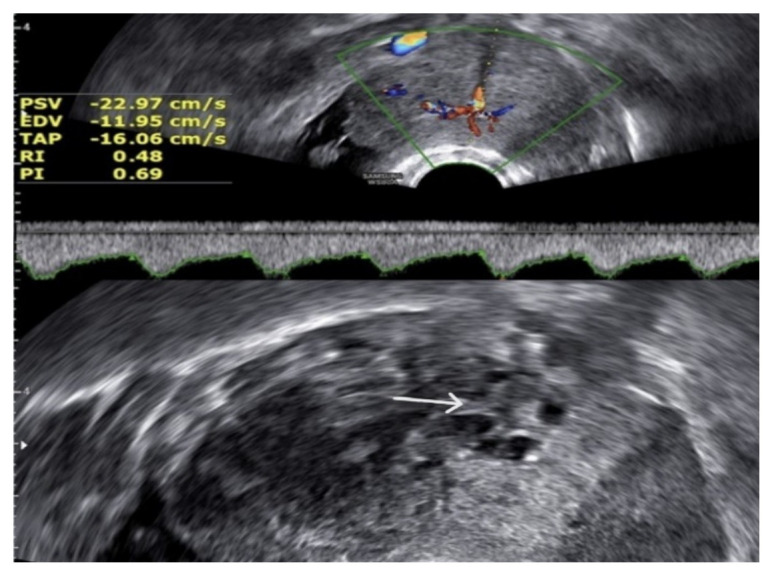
A 41-year-old gravida 1, para 1, female patient was admitted to our department for a laparoscopy because of a solid tumor mass positioned near the left ovary (ovary marked with arrow), diagnosed 18 months before admission during routine gynecological examination. Regular transvaginal ultrasound examinations were performed, which showed a slow but steady increase in the size of the solid tumor mass. From the fist to the last ultrasound examination, it increased in diameter from 3 cm to 5 cm in size with central vascularization and was positioned near the left ovary, but it did not incorporate it. The pulsatility index (PI) was 0.69 and the resistance index (RI) was 0.48. Tumor marker levels were not elevated (CEA 1.08 µg/L, CA19-9 < 2 kIU/L, CA125 26.8 kIU/L), and the patient was not experiencing any symptoms.
